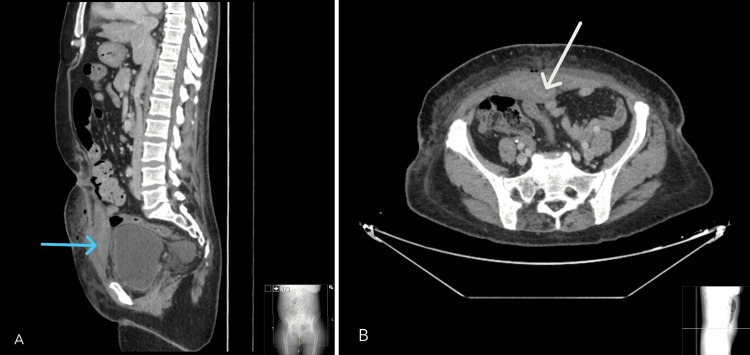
Abdominal CT scan: sagittal view (A) shows a fluid collection arising from the right rectus abdominis muscle, consistent with a hematoma (blue arrow), and demonstrating a partially organized wall suggesting abscess formation (axial view (B), white arrow). CT - computed tomography
